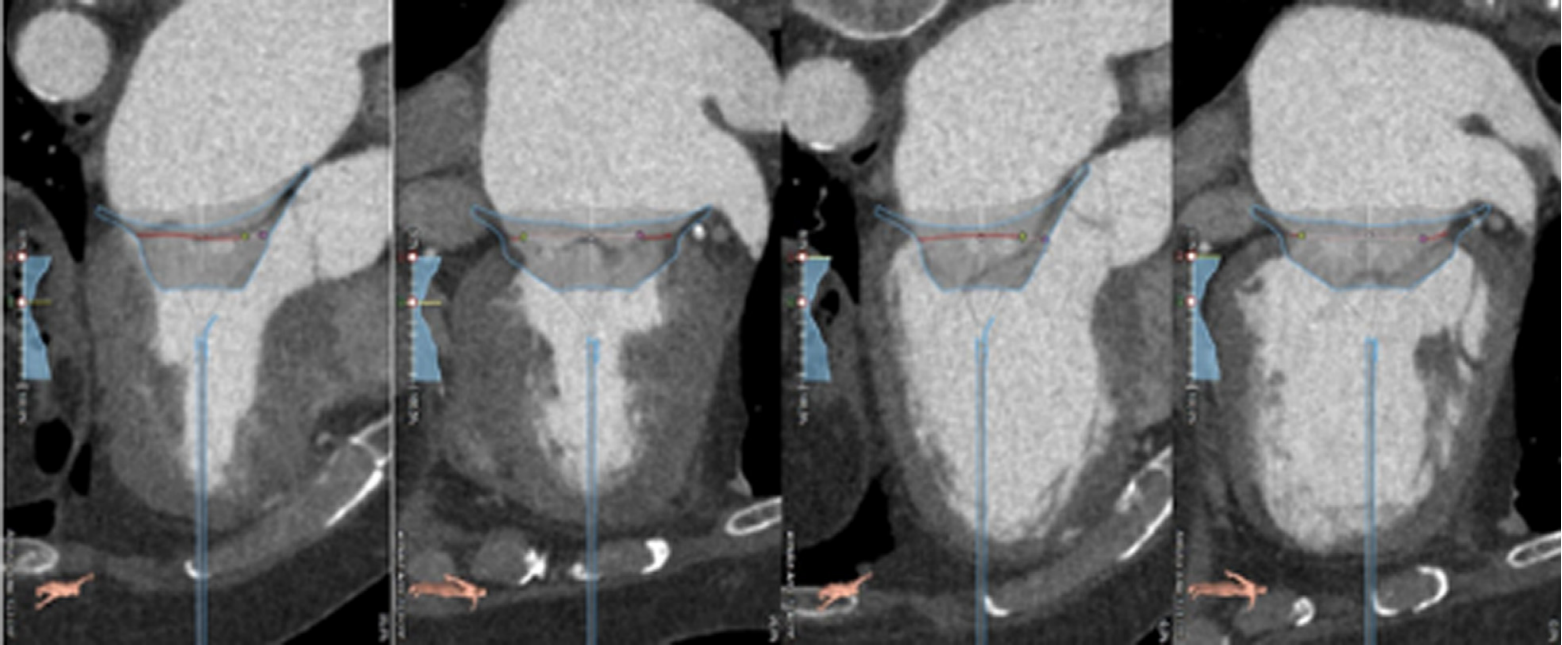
Cardiac Computed Tomography Radiologic study of the position of the Valve 37M LP during systole and diastole.
